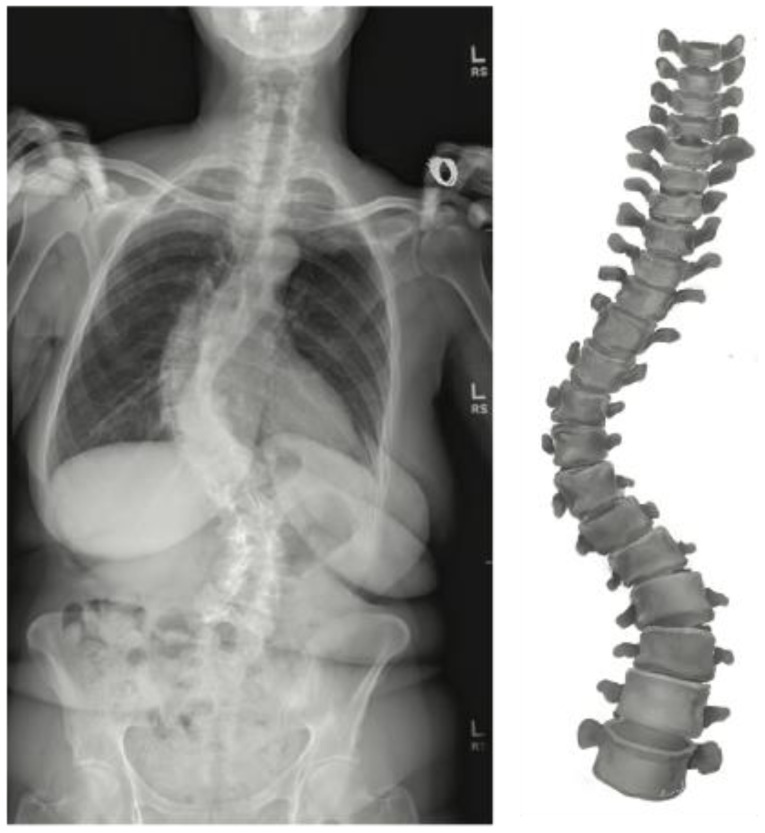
Generation of three-dimensional spinal models for pre-operative planning. Radiographic views can be integrated to create a virtual model that can be examined and manipulated.
